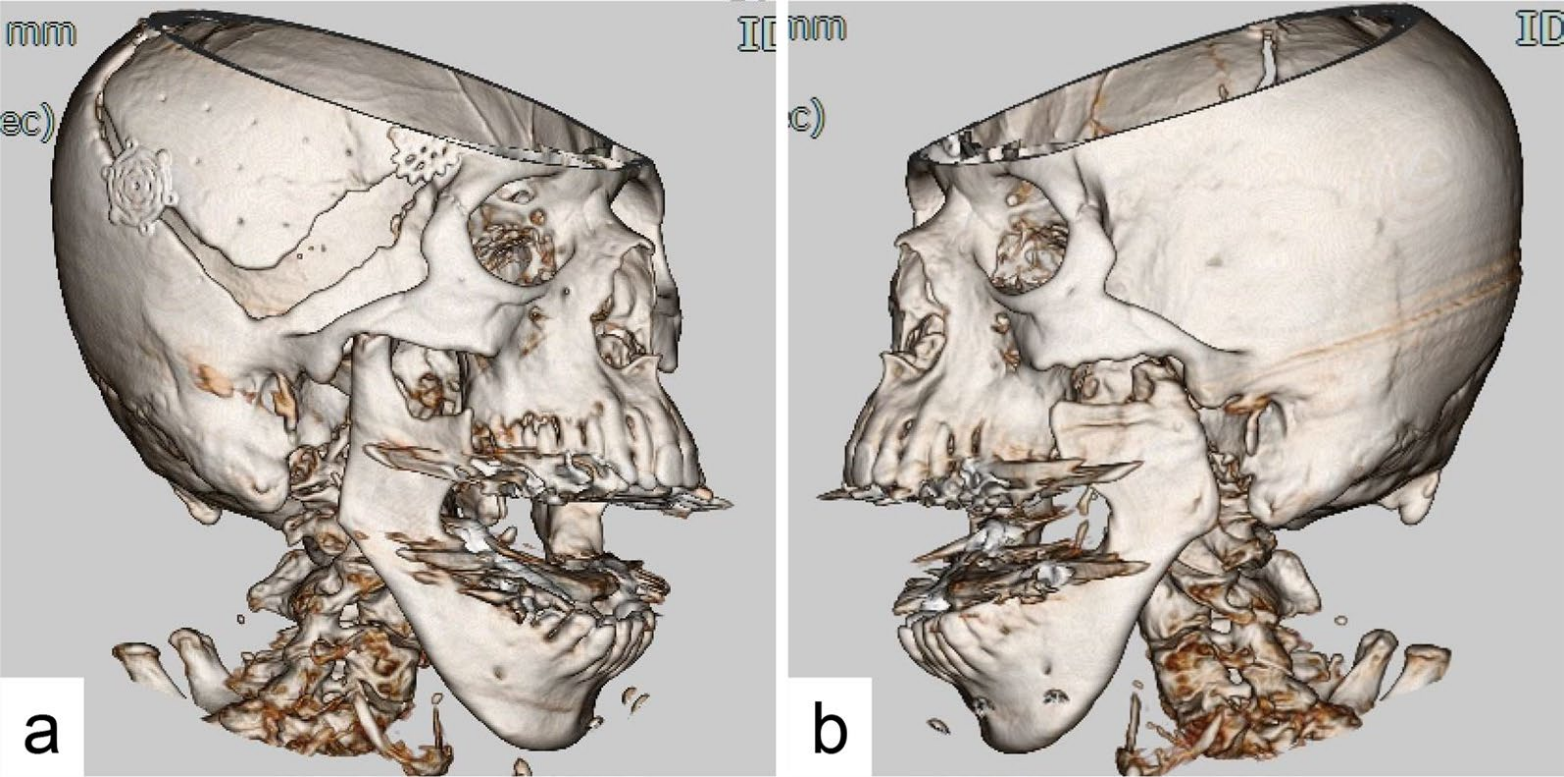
Computed tomography at pretreatment. The left condylar process fracture on the medial side. Bilateral mandibular condyles greatly exceeded the articular tubercle. After craniotomy operation of the temporal region at right side. a Right side; b left side
